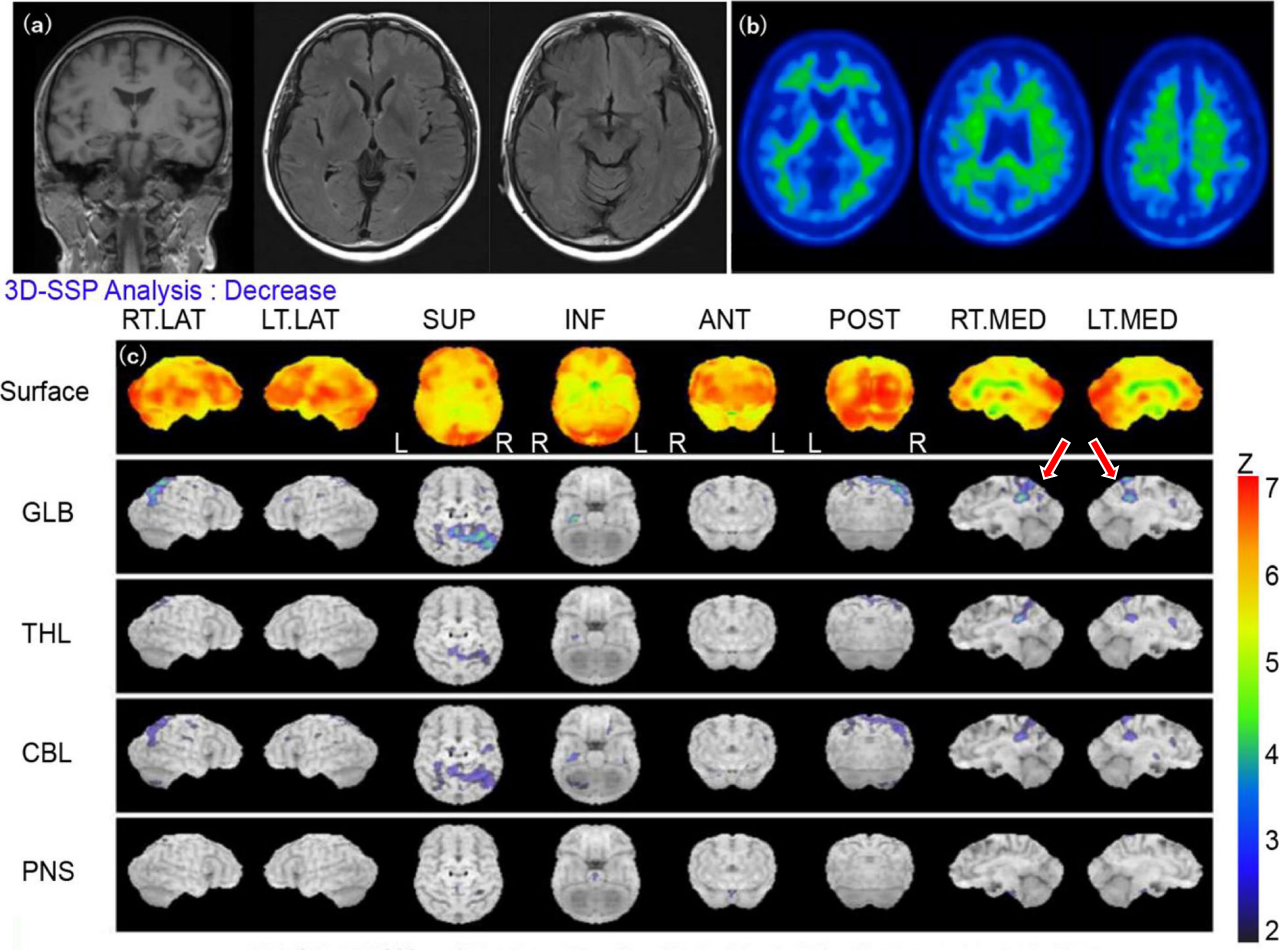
Brain imaging results of the patient with late-onset ADHD. a No particular change in brain MRI. b Negative finding of amyloid deposition in PiB-PET. c Slightly decreased blood flow in the posterior cingulate cortex on SPECT (contrast substance:^123^I-IMP). Abbreviations: ADHD, attention deficit hyperactivity disorder; ^123^I-IMP, N-isopropyl iodo-amphetamine; MRI, magnetic resonance imaging; PiB-PET, Pittsburgh compound B positron emission tomography; SPECT, single-photon emission computed tomography
